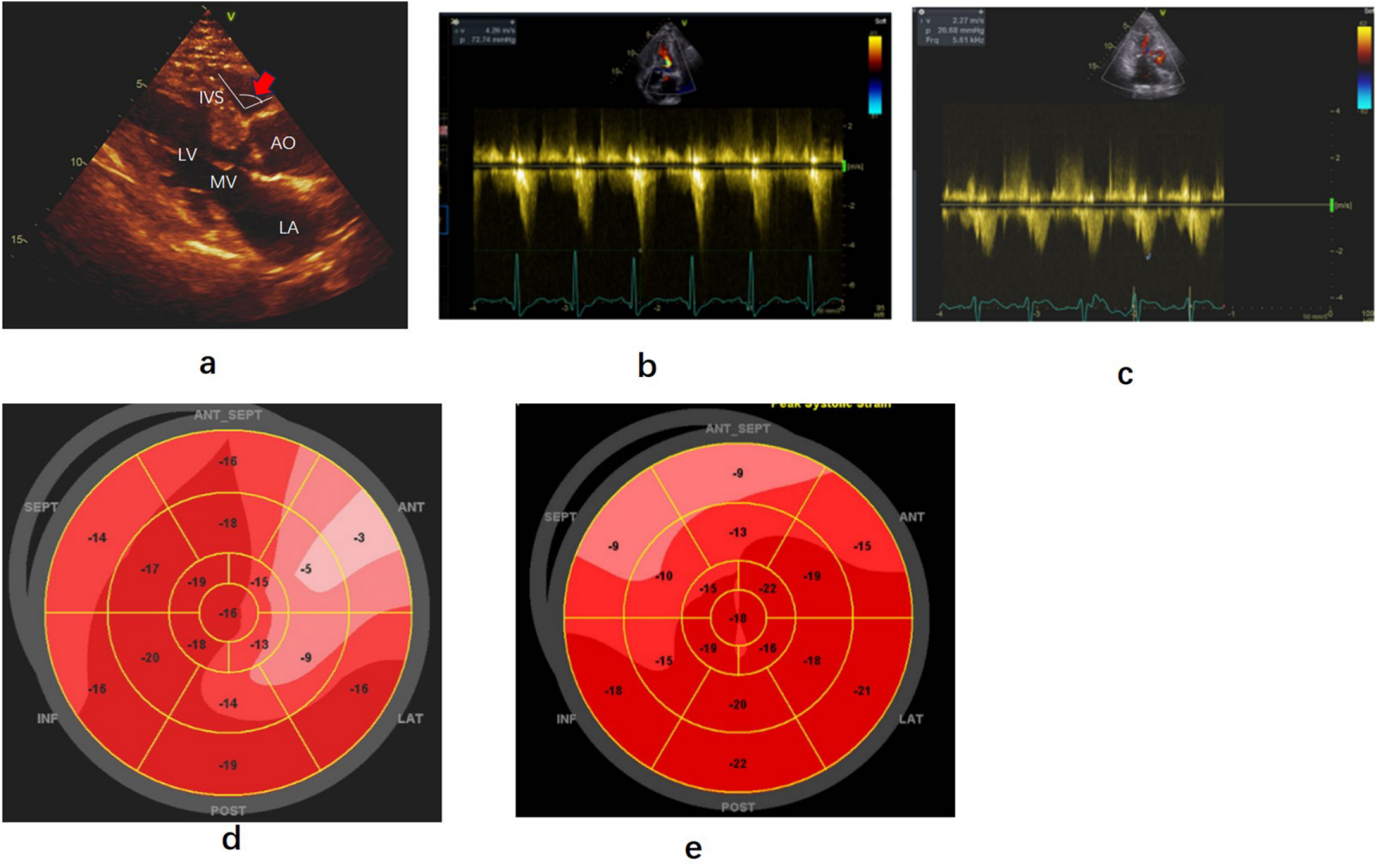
Echocardiography findings before and after precedure. (a) Parasternal long-axis view of echocardiography showing hypertrophy of the base of the left ventricular septum and the angle between the aorta and right ventricular interventricular septum(red arrow). (b,c) Left ventricular outflow gradient provoked by dobutamine before and after radiofrequency ablation. (d,e) Strain bullseye plotsduring baselineand three months after dobutamine stress post-ablation. RA, right atrium; RV, right ventricle; LV, left ventricle; LA, left atrium; AO, Aorta; AV, aortic valve; MV, mitral valve; SEPT, posterior septum; ANT-SEPT, anterior septum.
